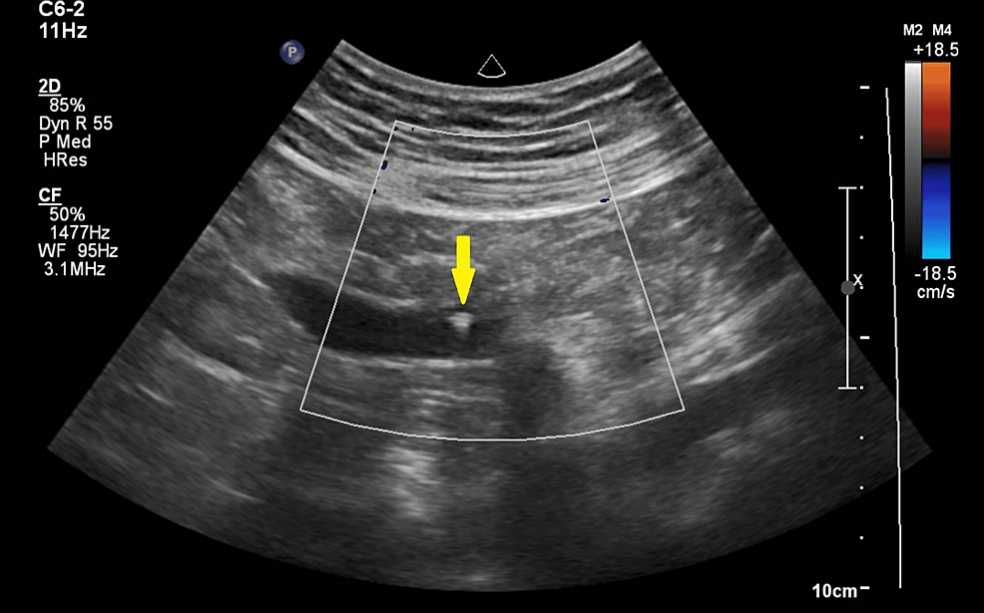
Image of the appendix during the abdominal ultrasound the yellow arrow indicates the appendicolith.
